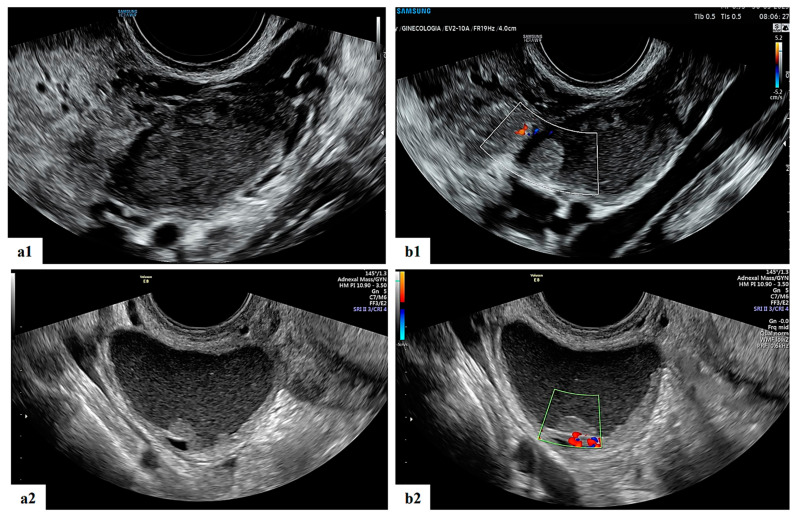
Grayscale (a1,a2) and color Doppler (b1,b2) ultrasound images of two decidualized endometriomas in our series (ongoing patients): moderately vascularized internal cyst wall, rounded papillary projections with smooth contours and cyst content with ground-glass echogenicity.
